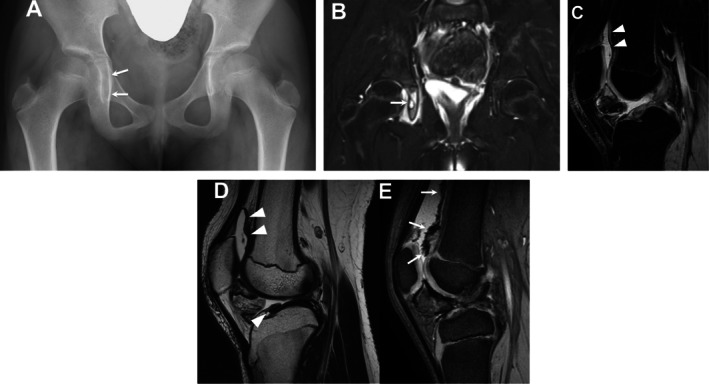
Differential diagnoses of JIA. (A, B) Osteoid osteoma of the right ischium in a 13‐year‐old girl with right hip pain. Radiograph of the hip (A) showed mild juxta‐articular osteopenia and enlargement of the acetabular teardrop (arrows). Fat‐suppressed T2‐weighted image (B) showed joint effusion, intraosseous and juxtaosseous edema of the acetabular teardrop (inferomedial aspect of the acetabulum), and a nidus of osteoid osteoma, seen as a well‐defined, high intensity focus surrounded by a low intensity rim (arrow). (C, D) Hemophilic arthropathy in a 13‐year‐old boy with painful knee swelling. Hemosiderin deposition along the synovial lining (arrowheads) was more clearly depicted on gradient‐echo T2‐weighted image (C) than on the spin echo T2‐weighted image (D). (E) Tenosynovial giant cell tumor in a 9‐year‐old boy with knee swelling. Hemosiderin deposition was depicted as low signal intensity with “blooming” on T2* gradient‐echo sequence (arrows).
